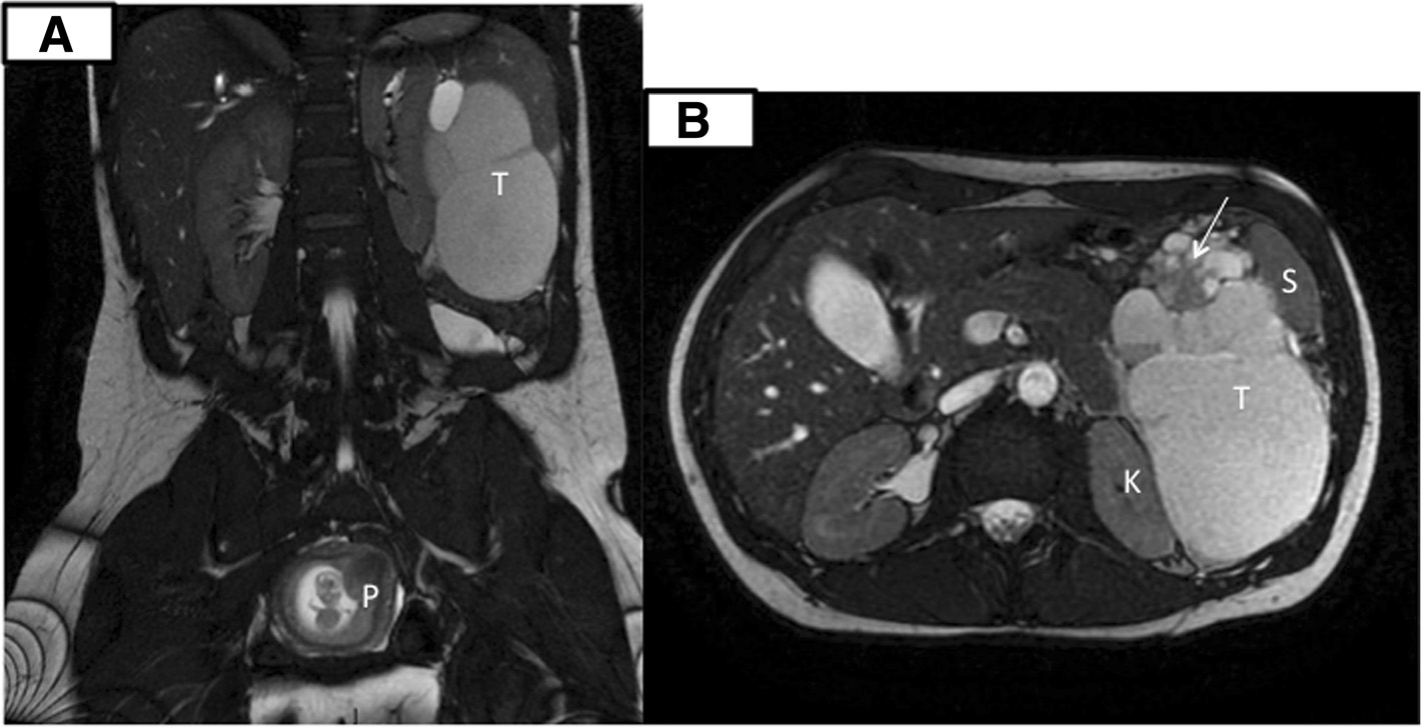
Coronal (a) and transversal (b) MRI T2 (FIESTA) view. Large tumor (T) medial to the spleen (S) and adjacent to the pancreatic tail dislocating the left kidney (K) medial and cranial. Mostly high signal intensity at T2 and intermediate to high at T1, indicating cystic components with protein or blood. A smaller part of the lesion had lower signal and diffusion restriction indicating more solid parts. (P) is the pregnant uterus. A solid pseudopapillary epithel neoplasia (SPEN) of the pancreas was suspected in this young woman with an encapsulated lesion with cystic, solid and hemorrhagic components
